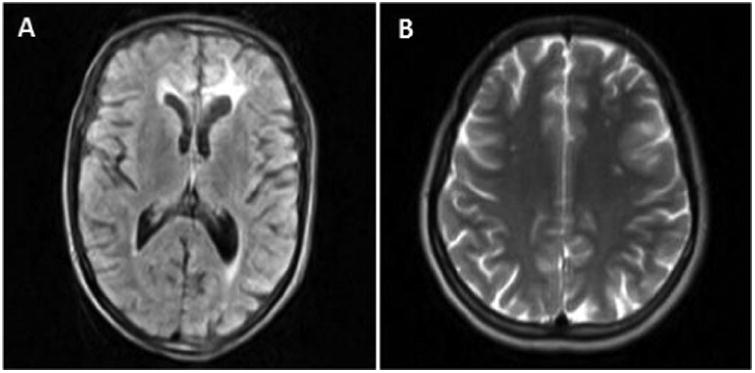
Brain MRI. Brain MRI of V-29 shows white matter hypersignal changes in FLAIR (A) and T2-weighted images (B), especially in bilateral fronto-parietal, periventricular, and centrum semiovale areas.
